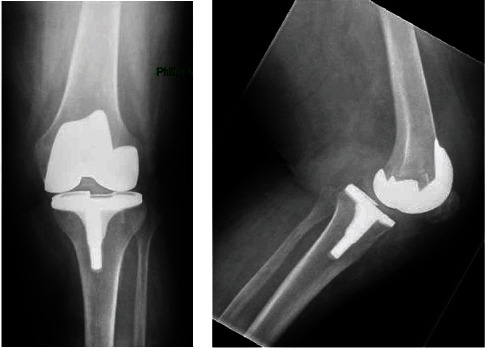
(a) Anteroposterior radiograph of case 3 at presentation in the emergency room. (b) Lateral radiograph of case 3 at presentation in the emergency room.
